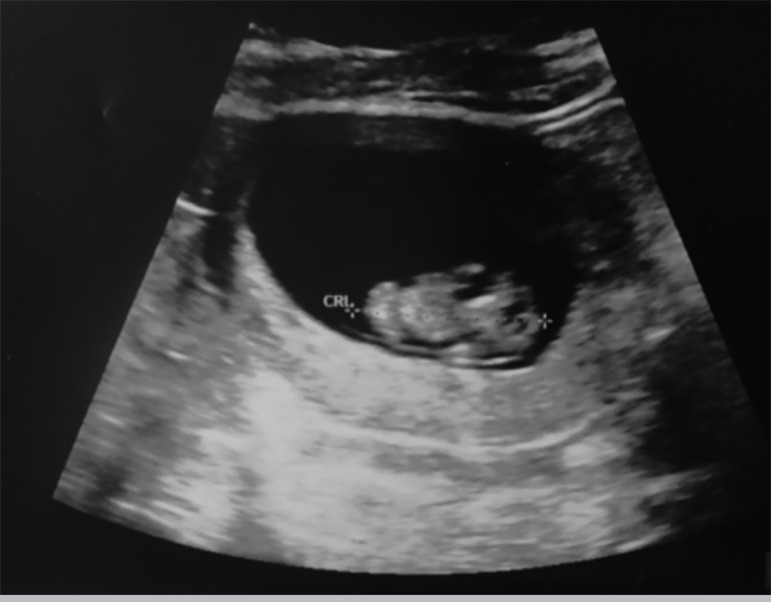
Picture shows the ultrasound scan performed at 10 weeks of gestation
